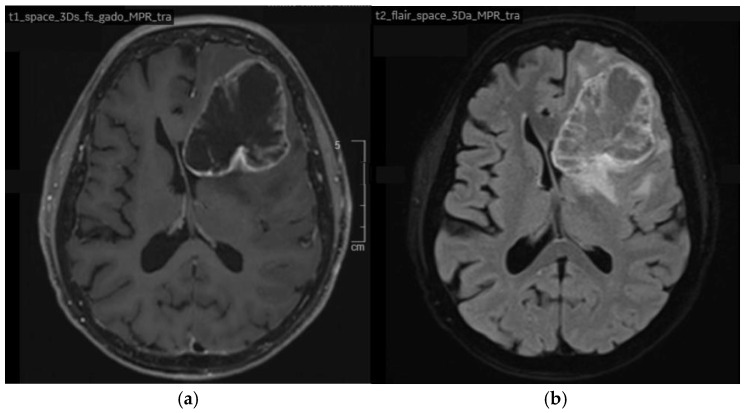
Axial brain magnetic resonance imaging (MRI) showing an isolated left frontal metastasis: (a) T1-weighted sequence after gadolinium administration; (b) T2-weighted FLAIR sequence.
